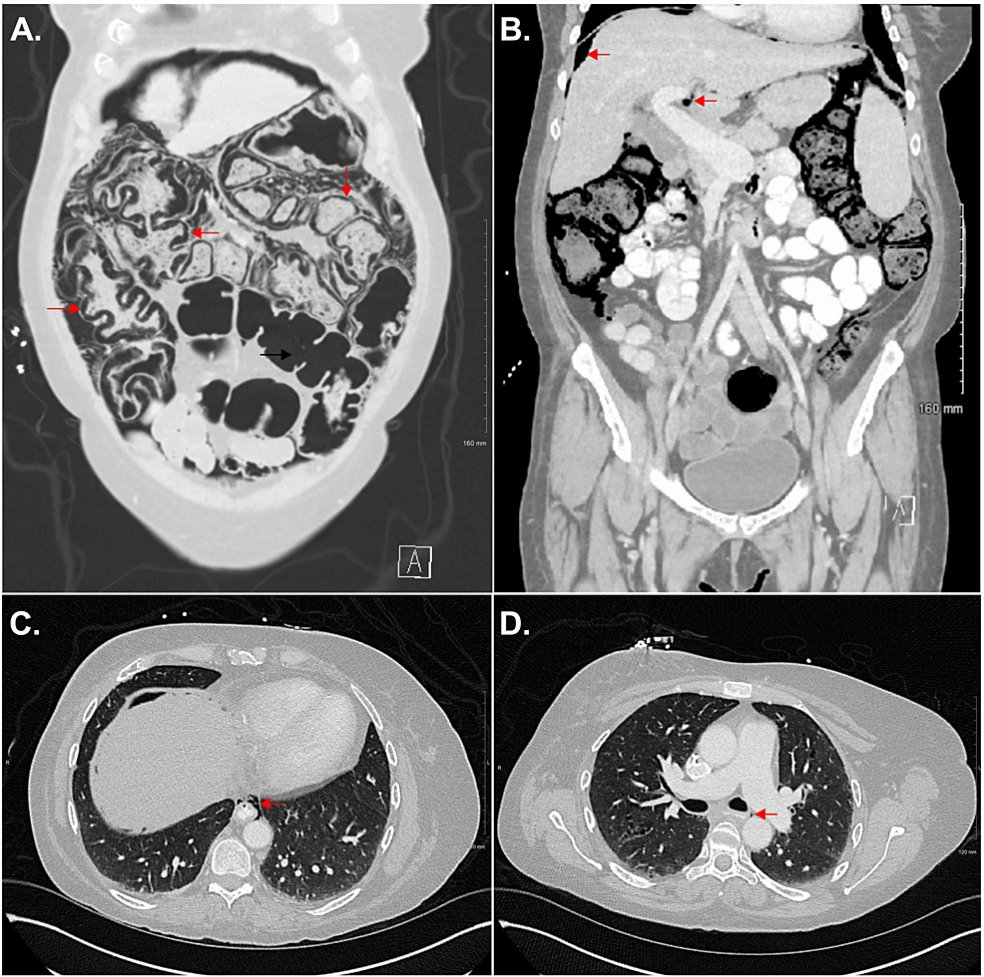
Transverse and coronal CT images showing pneumatosis intestinalis, pneumobilia, pneumomediastinum, and pneumoperitoneum (A) Coronal CT showing massive pneumatosis intestinalis (PI) in the ascending, transverse, and descending colon. Note the absence of PI in the sigmoid colon (represented by a single black arrow). (B) Coronal CT showing pneumoperitoneum (superior red arrow) and pneumobilia (inferior red arrow). (C) Transverse CT image showing pneumomediastinum at the level of the diaphragm (red arrow). (D) Transverse CT image showing pneumomediastinum less prominent more superiorly, just below the level of the carina (red arrow).
